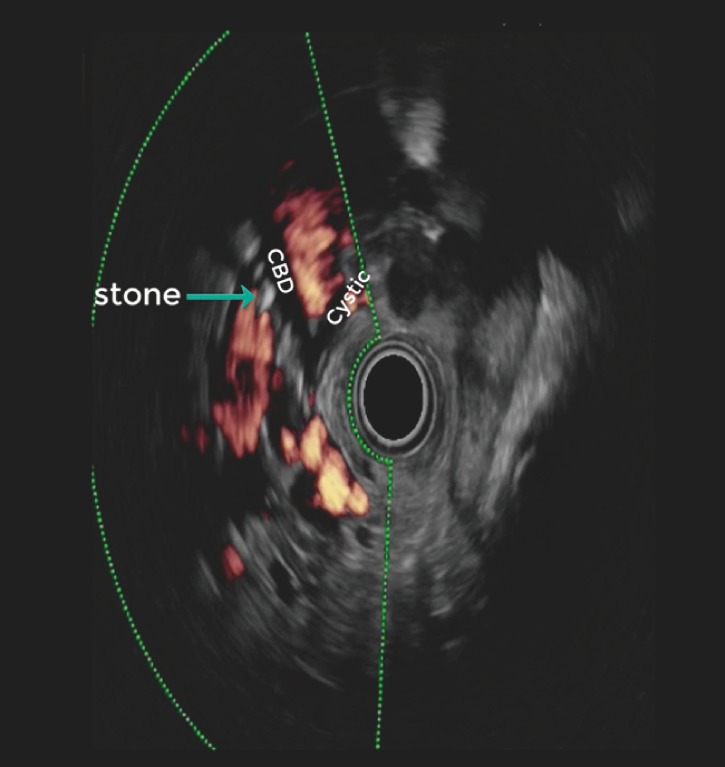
Endoscopic ultrasound image showing the main bile duct and cystic duct, with a gallstone within the common bile duct. Collateral vessels surrounding the biliary tract are also discernible. CBD, common bile duct.
